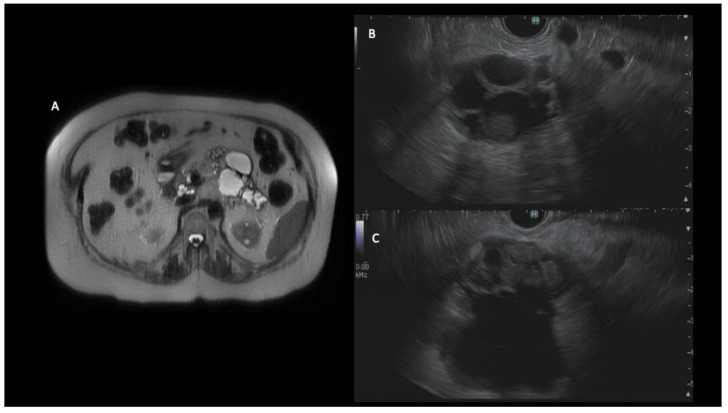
MRI of a 79-year-old female showed numerous cystic lesions with rapidly enlarging cysts in the distal body and tail of the pancreas (A). An endoscopic ultrasound demonstrated an approximately 50 mm cyst in the body of the pancreas associated with mural nodularity and pancreatic ductal dilation up to 20 mm (B,C). A fine-needle biopsy was obtained and showed evidence of at least high-grade dysplasia. The patient underwent distal pancreatectomy and was diagnosed with invasive moderately differentiated adenocarcinoma (T3N0M0).
